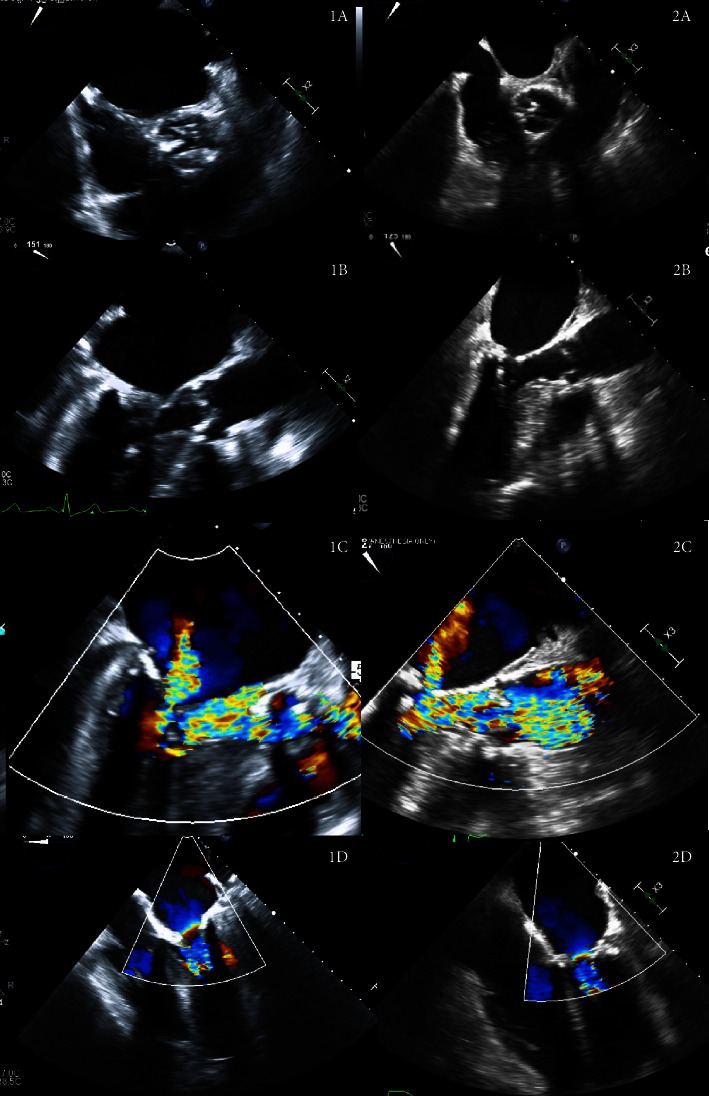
Pre-cardiopulmonary bypass transesophageal echocardiographic views for Patient 1 (1A–1D) and Patient 2 (2A–2D). A: midesophageal aortic valve short-axis view. B: midesophageal long-axis view during systole with SAM. C: midesophageal long-axis view during systole with SAM and color-flow Doppler. D: midesophageal four-chamber view with color-flow Doppler.
